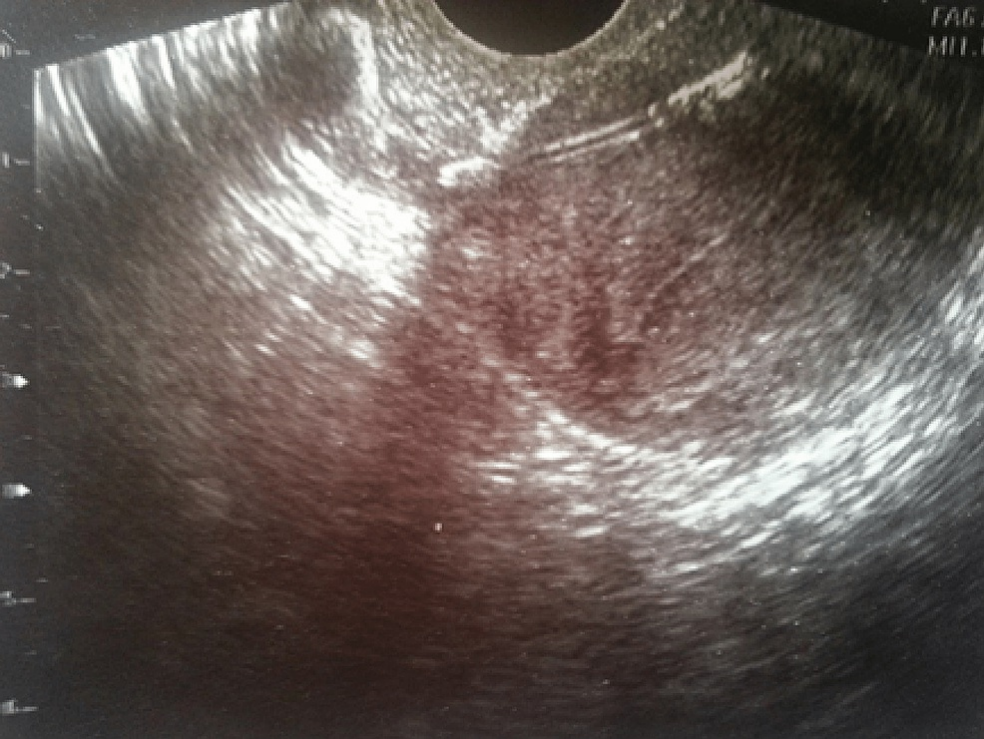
TVS with empty bladder showed IUCD to be embedded through the myometrium of the anterior uterine wall TVS: transvaginal ultrasound; IUCD: intrauterine contraceptive device
